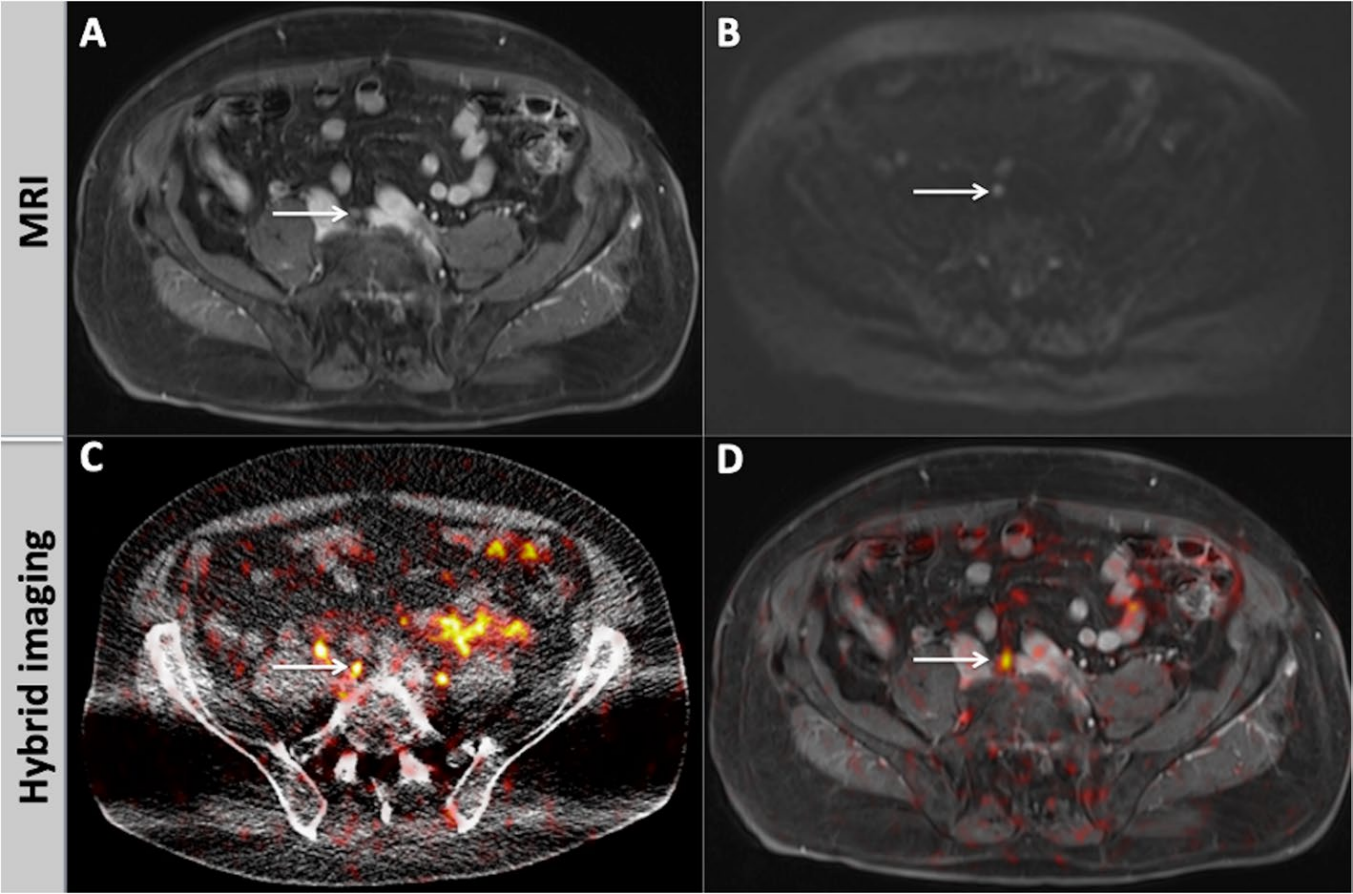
A 76-year old patient with biochemical recurrence of PCa referred to our institution for imaging. His PSA level at the time of examination was 5.0 ng/ml. Contrast enhanced-T1w fatsat (A) and DWI (b800; B) at the pelvic level merely show a small (5 mm), oval, presacral lymph node (arrow), which was not considered suspicious. In the fused hybrid modalities PET-CT (C) and PET-MRI (D), however, the above-mentioned lymph node shows a focal, intensive [^68^Ga]PSMA-11 uptake, suggestive of metastatic involvement (arrow). In PET-CT (C), both ureters are visible laterally of the mentioned lymph node
